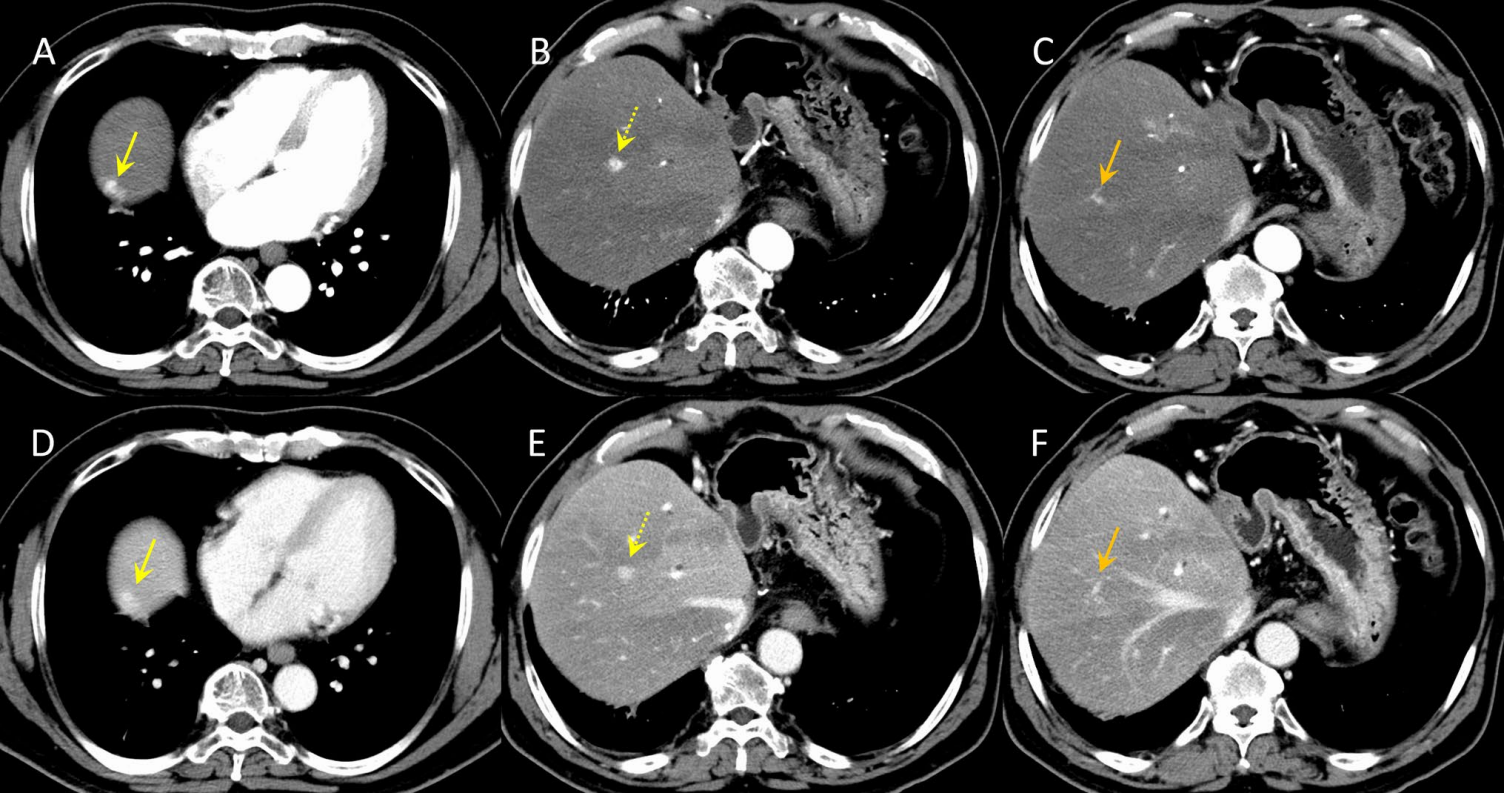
Contrast-enhanced computed tomography images of focal nodular hyperplasia one year after liver transplantation. Three lesions were hyperattenuating in the arterial phase (A: dome, yellow arrow, B: S8, dotted yellow arrow, C: S8/7, orange arrow). These lesions remained hyperattenuating in the portal venous phase (D: dome, yellow arrow, E: S8, dotted yellow arrow, F: S8/7, orange arrow)
